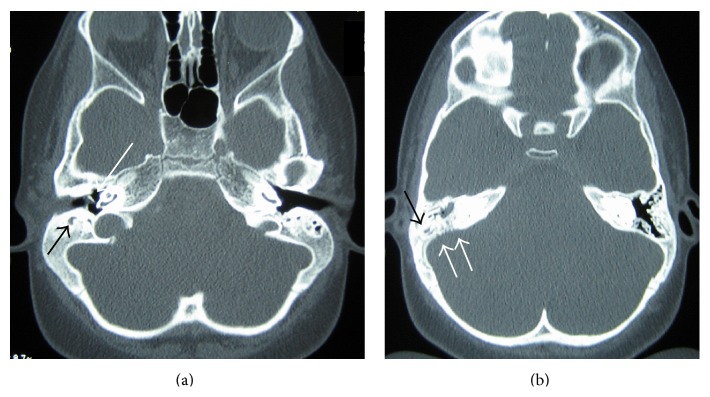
(a) and (b), axial sections from the preoperative temporal bone CT (without contrast) of the presented case. (a) Section through the mesotympanum showing the poorly developed mastoids on both sides. The right mastoid cells are filled with soft tissue (short arrow), while the middle ear cavity is free of effusion (long arrow). (b) Section at the level where the bone erosion can be observed (double arrows). Again, the right mastoid air cells and antrum are cloudy (short arrow).
